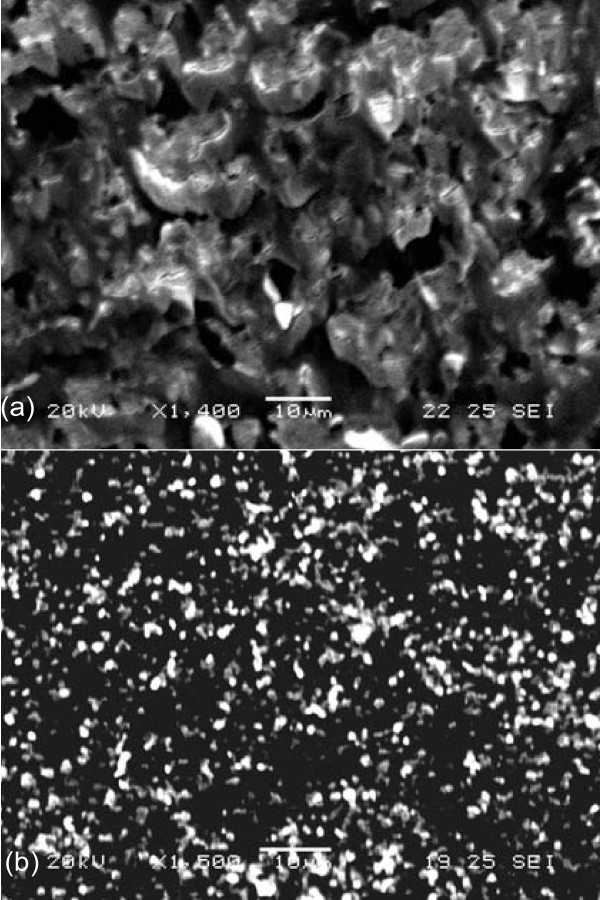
SEM microstructure of the dried Al_2_O_3_-water nanofluids before re-ultrasonication (a) and after re-ultrasonication (b).
